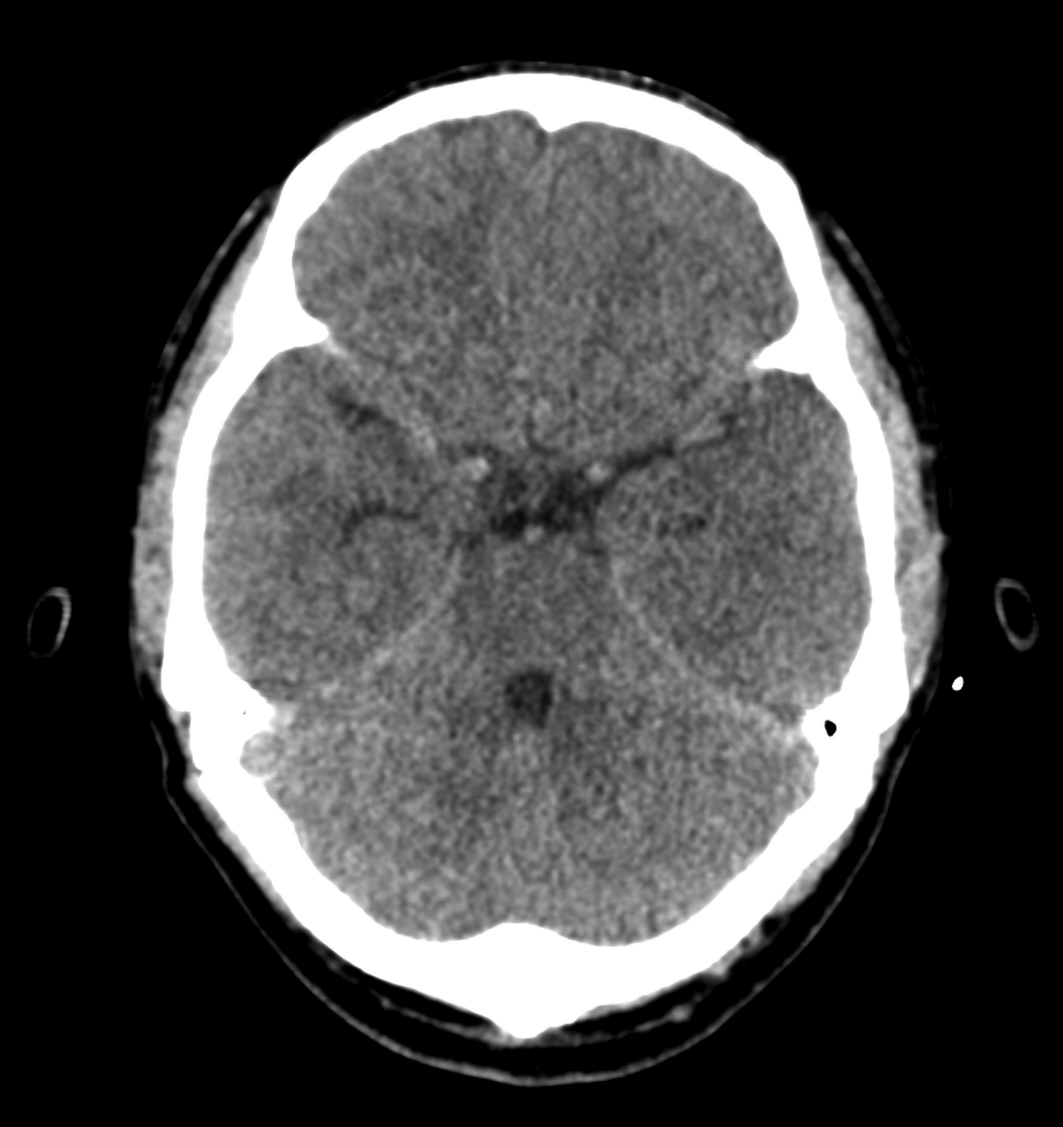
CT scan of the head showing cerebral edema.
